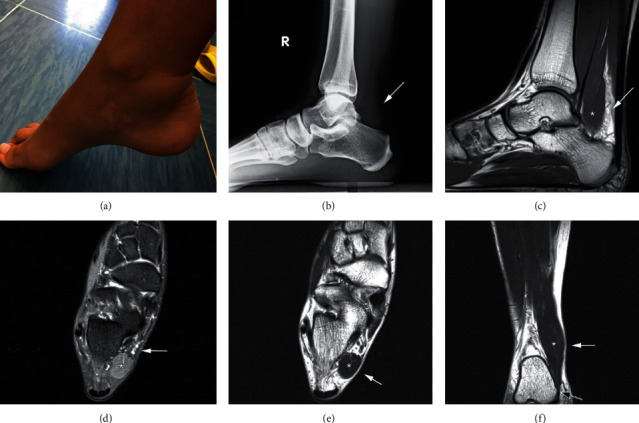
Preoperative images of the patient's right ankle presented in the first case. (a) A swelling is visible in the posteromedial part of the right ankle, which is more prominent in plantar flexion. (b) Obliteration of Kager's fat pad visible on the plain lateral radiograph of the right ankle is pointed by a white arrow. (c) T1-weighted sagittal MR image of the right ankle. Accessory soleus muscle (ASM) is marked with an asterisk (^∗^), and obliteration of the Kager's fat pad is pointed by a white arrow. (d) T2-weighted axial MR image of the right ankle. ASM is marked with an asterisk (^∗^), and neurovascular bundle in the posteromedial part of the ankle is pointed by a white arrow. (e) T1-weighted axial MR image of the right ankle. ASM is marked with an asterisk (^∗^), and elevation of the skin and subcutaneous tissue is pointed by a white arrow. (f) T1-weighted coronal MR image of the right ankle. ASM is marked with an asterisk (^∗^), the elevation of the skin and subcutaneous tissue is pointed by a white arrow, and muscular insertion of the ASM on the medial side of the calcaneus is pointed by a black arrow.
